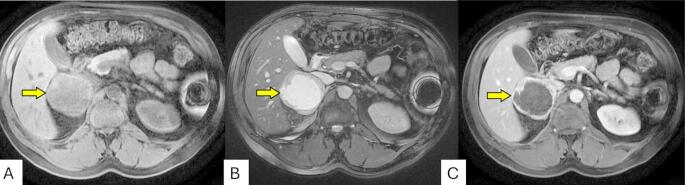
MRI of the abdomen showing a right adrenal mass (yellow arrows) in axial T1-weighted (A), T2-weighted (B), and post-contrast fat-suppressed T1-weighted (C) sequences. A T1-weighted image shows an isointense to mildly hypointense right adrenal lesion with well-defined margins. B T2-weighted image reveals marked hyperintensity (“light bulb sign”), a characteristic feature of pheochromocytoma. C Post-contrast T1-weighted image demonstrates peripheral enhancement with central necrosis
